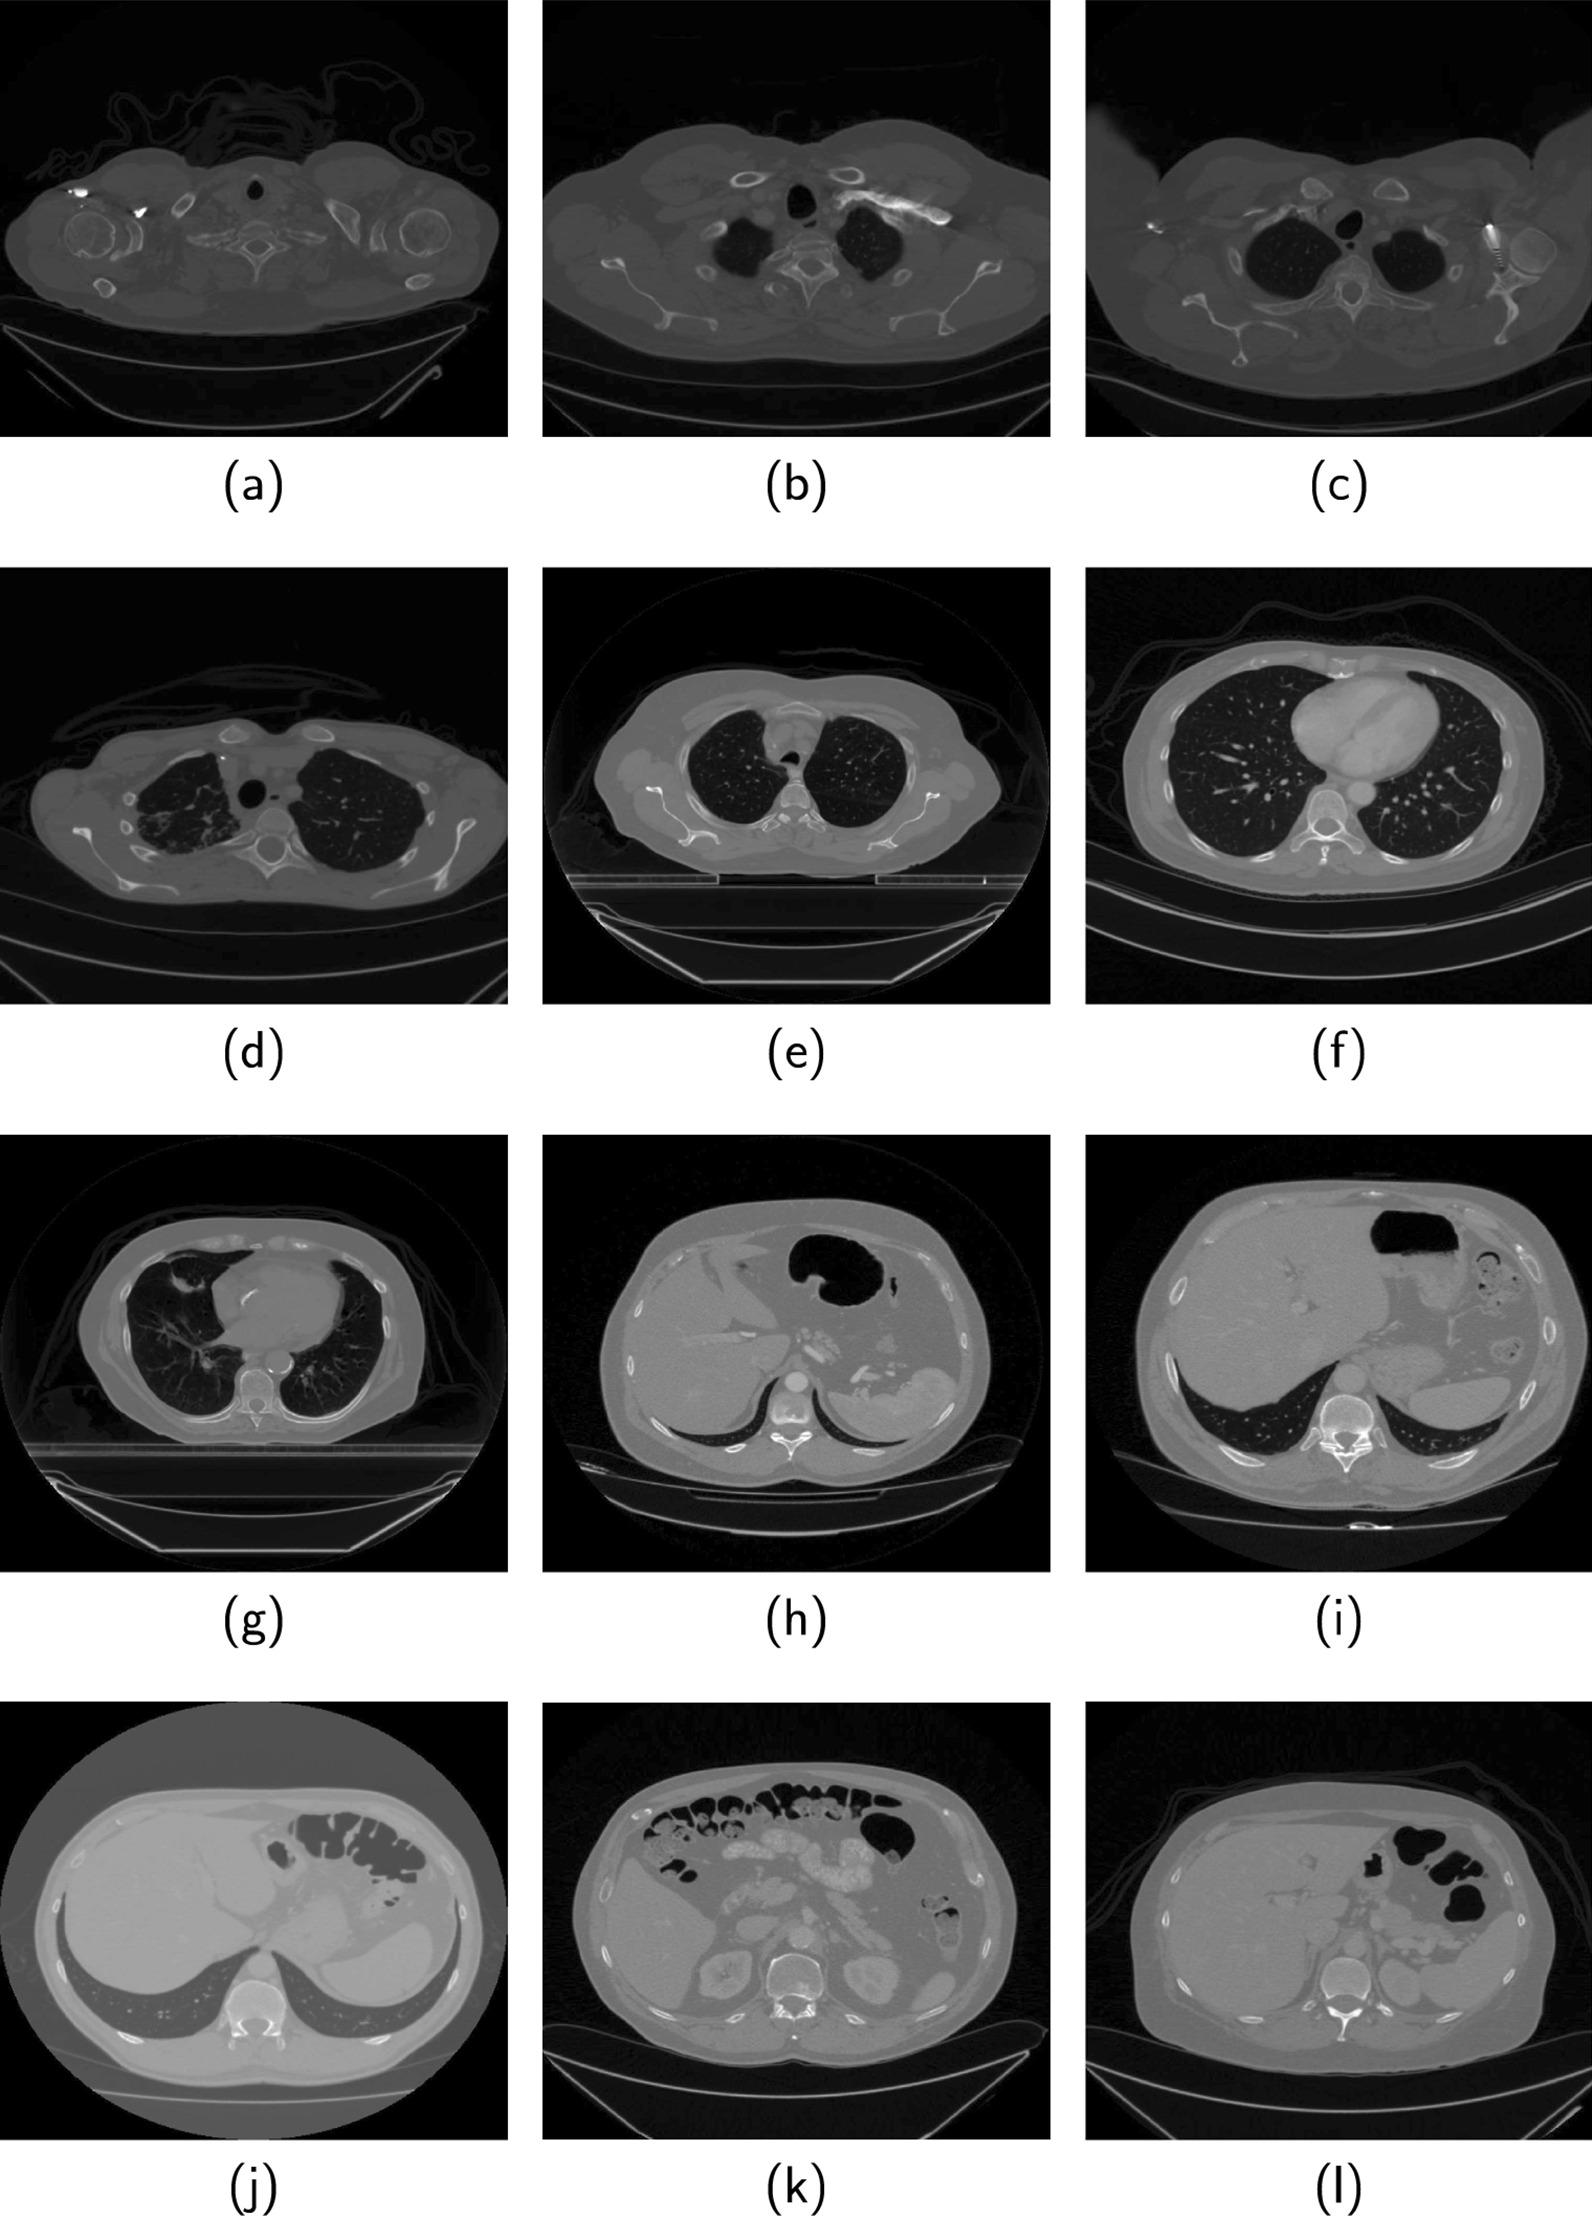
Examples of CT slices from [24, 25] that are acquired during CT lung screening. The slices are arranged successively such that (a) is the most superior and (l) is the most inferior within the thorax region. Slices in (a), (k) and (l) do not contain lung region
